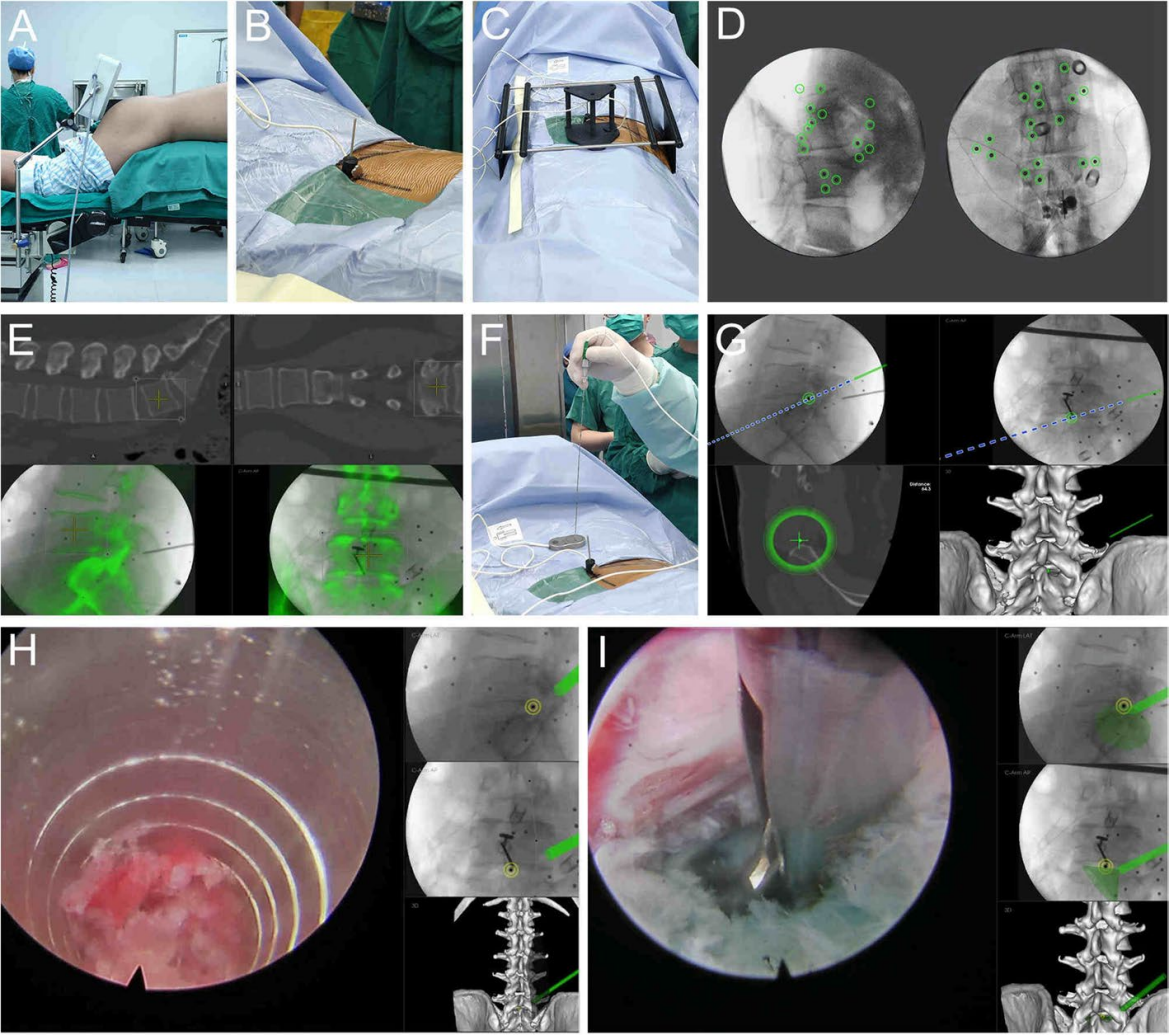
The procedure of PTED assisted by the EMN system. a The electromagnetic field generator was placed in the caudal site of the surgical area. b The patient tracker was fixed on the adjacent spinous process of the operative level. c The mapper bridge was placed in the surgical area. d The fluoroscopy images were transmitted to the EMN system for automatic registration. e The intraoperative two-dimensional fluoroscopy images were used to match the preoperative three-dimensional CT images. f Surgical instruments were registered. g The percutaneous puncture was performed under EMN guidance. h The foraminoplasty was done under the surveillance of navigation and endoscope. i Discectomy was performed under EMN guidance. PTED, percutaneous transforaminal endoscopic discectomy; EMN, electromagnetic navigation
